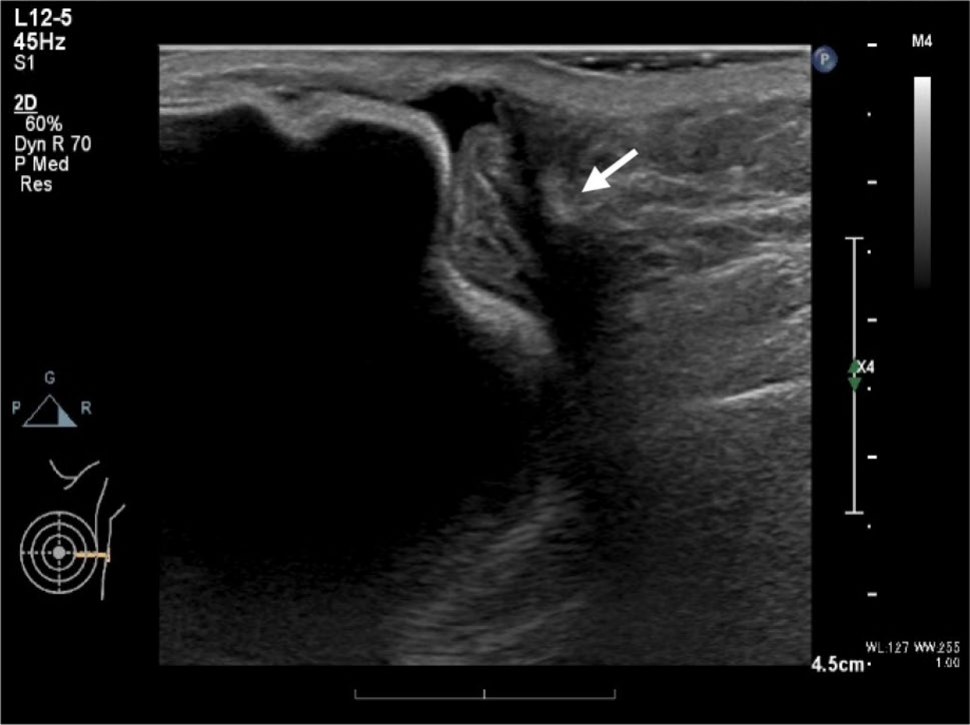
Ultrasound demonstrates fluid collection and suspended solids around the SBI with rippled shell
